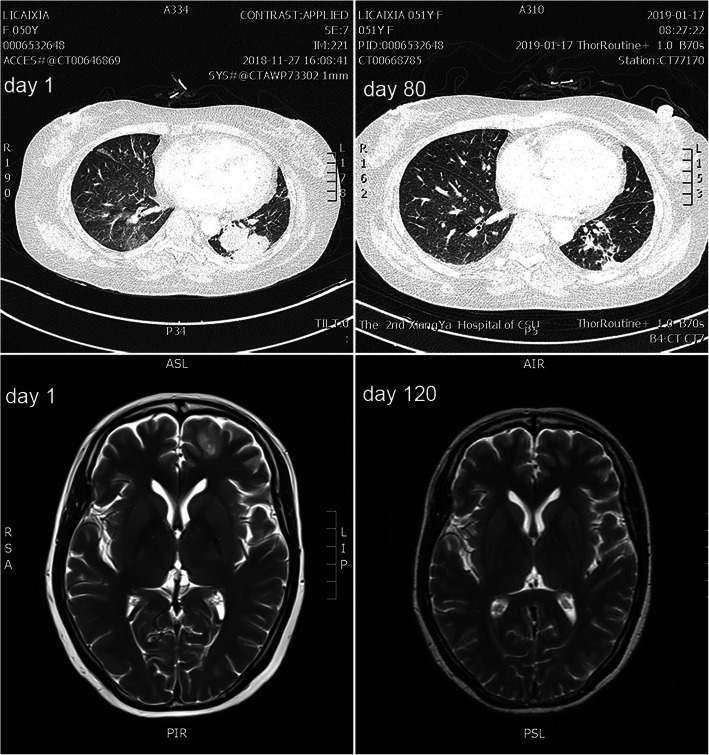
Lung CT and head MRI images of No.7 patient
